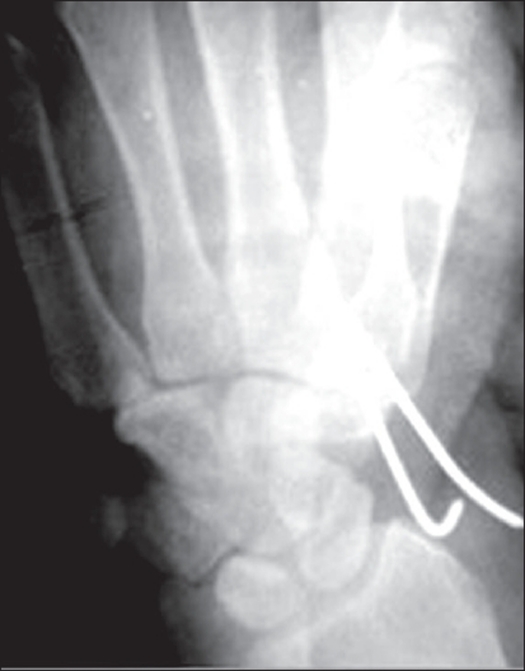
Postoperative X-ray of the wrist (A.P.) showing trapezoid fracture stabilized wirh Kirschner wire
